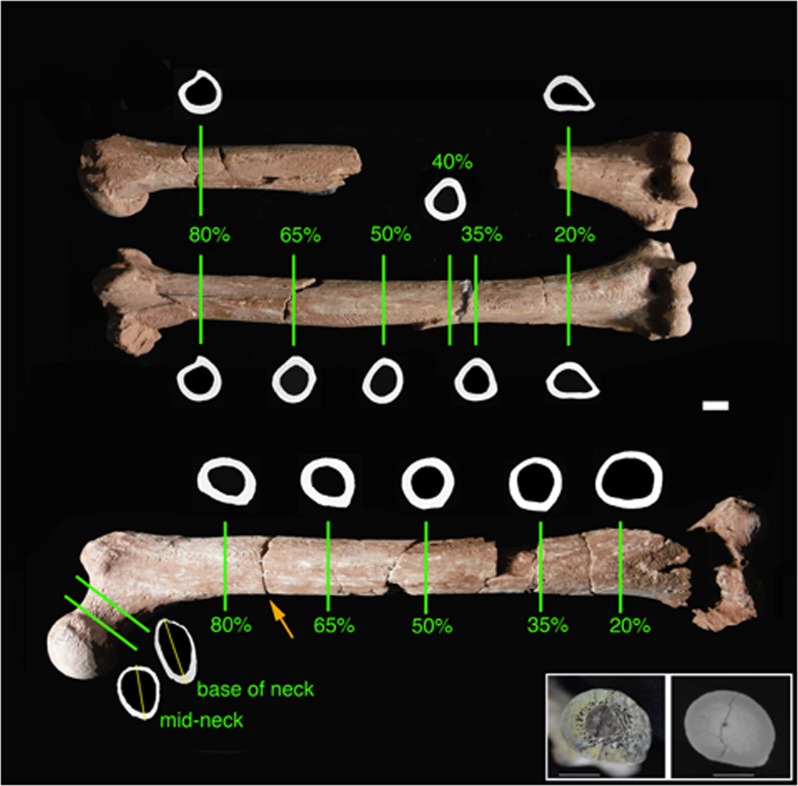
Section locations and cortical bone cross-sectional outlines for A.L. 288–1 humeri and femur, determined from CT scans. For diaphyseal sections, medial is to the left, anterior above; for femoral neck sections, anterior is to the left, superior above (medial and lateral orientations of (left) femur and left humerus reversed for consistency). Yellow lines through femoral neck sections indicate planes where superior and inferior cortical thicknesses were measured (as in [14]). Inset at lower right shows physical section (left) and CT image at an adjacent location (right) for a natural break in the femur at about 75% of length', indicated with an orange arrow in the main figure. Scale bars are 10 mm.
